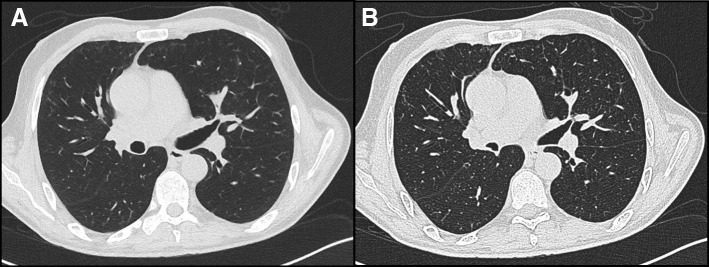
Two slices from computed tomography imaging of the chest approximately three weeks after re-presentation demonstrating with image a on the left performed at full inspiration and image b on the right performed at full expiration. The images demonstrate some mild improvement in the bronchial wall thickening affecting the left upper lobe bronchus seen in image 4 but, more importantly, little change in lung lucency on expiration due to significant homogenous gas trapping
